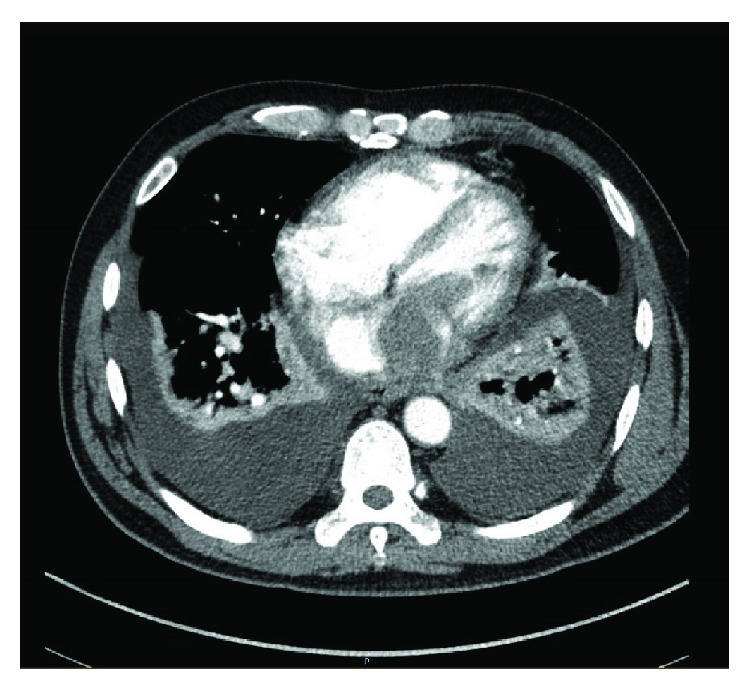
CT angiogram displayed moderate bilateral pleural effusions, a mass in the right ventricle, and a mass invading into the left atrium through the mitral valve into the left ventricle.
